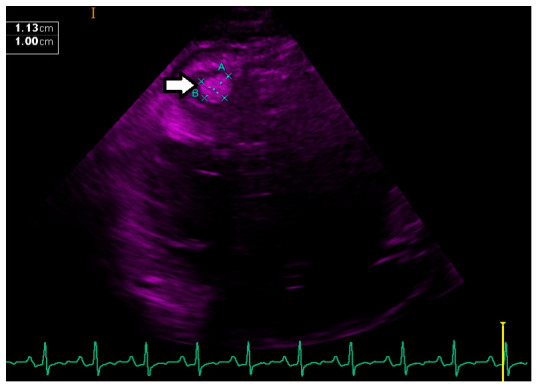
Echocardiogram (Apical 4 chamber view) showing dilated right ventricle with apical thrombus measurements.
